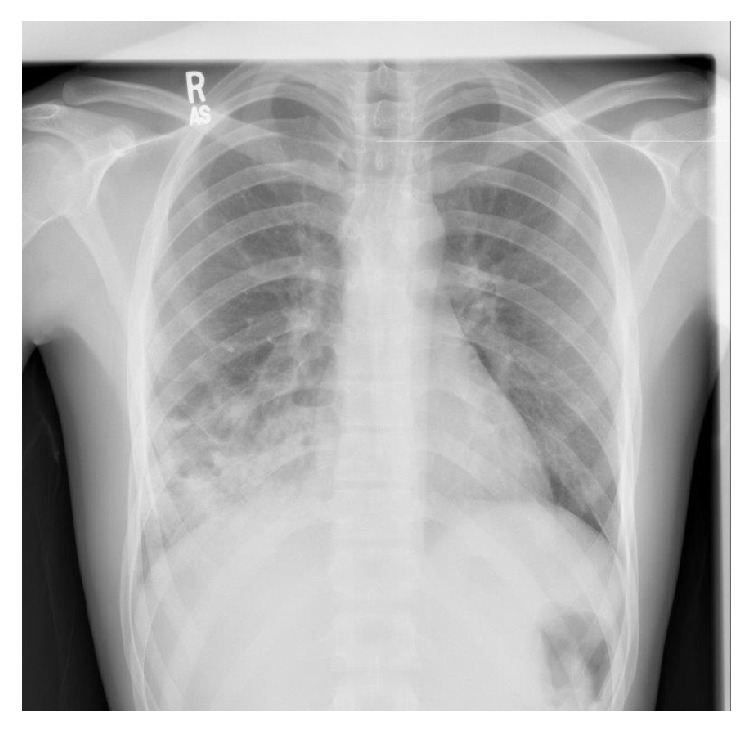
Chest X-ray obtained 2 hours after the trauma showing multiple cystic lesions containing air-fluid levels.
